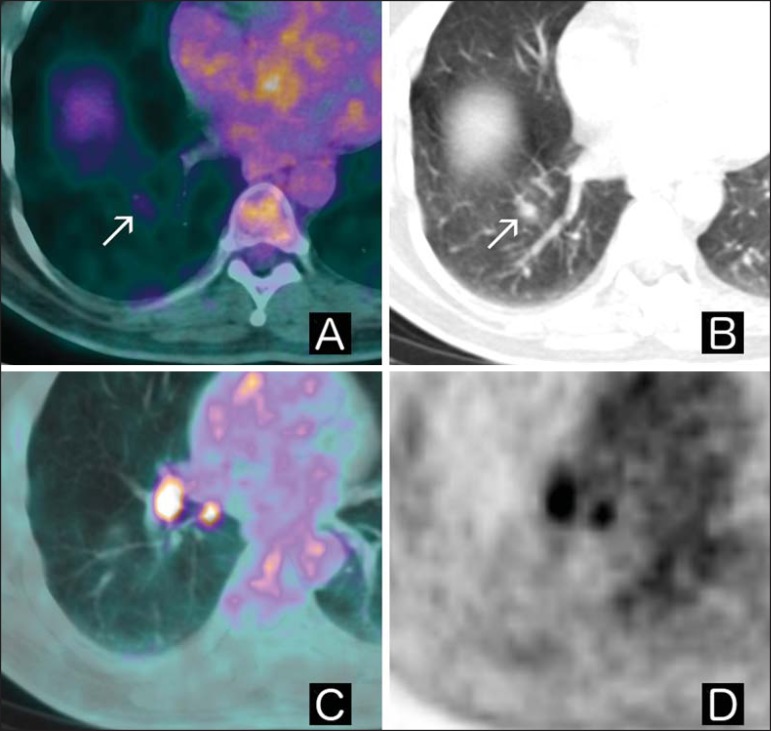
A: Axial fused ^18^F-FDG PET/CT image.
